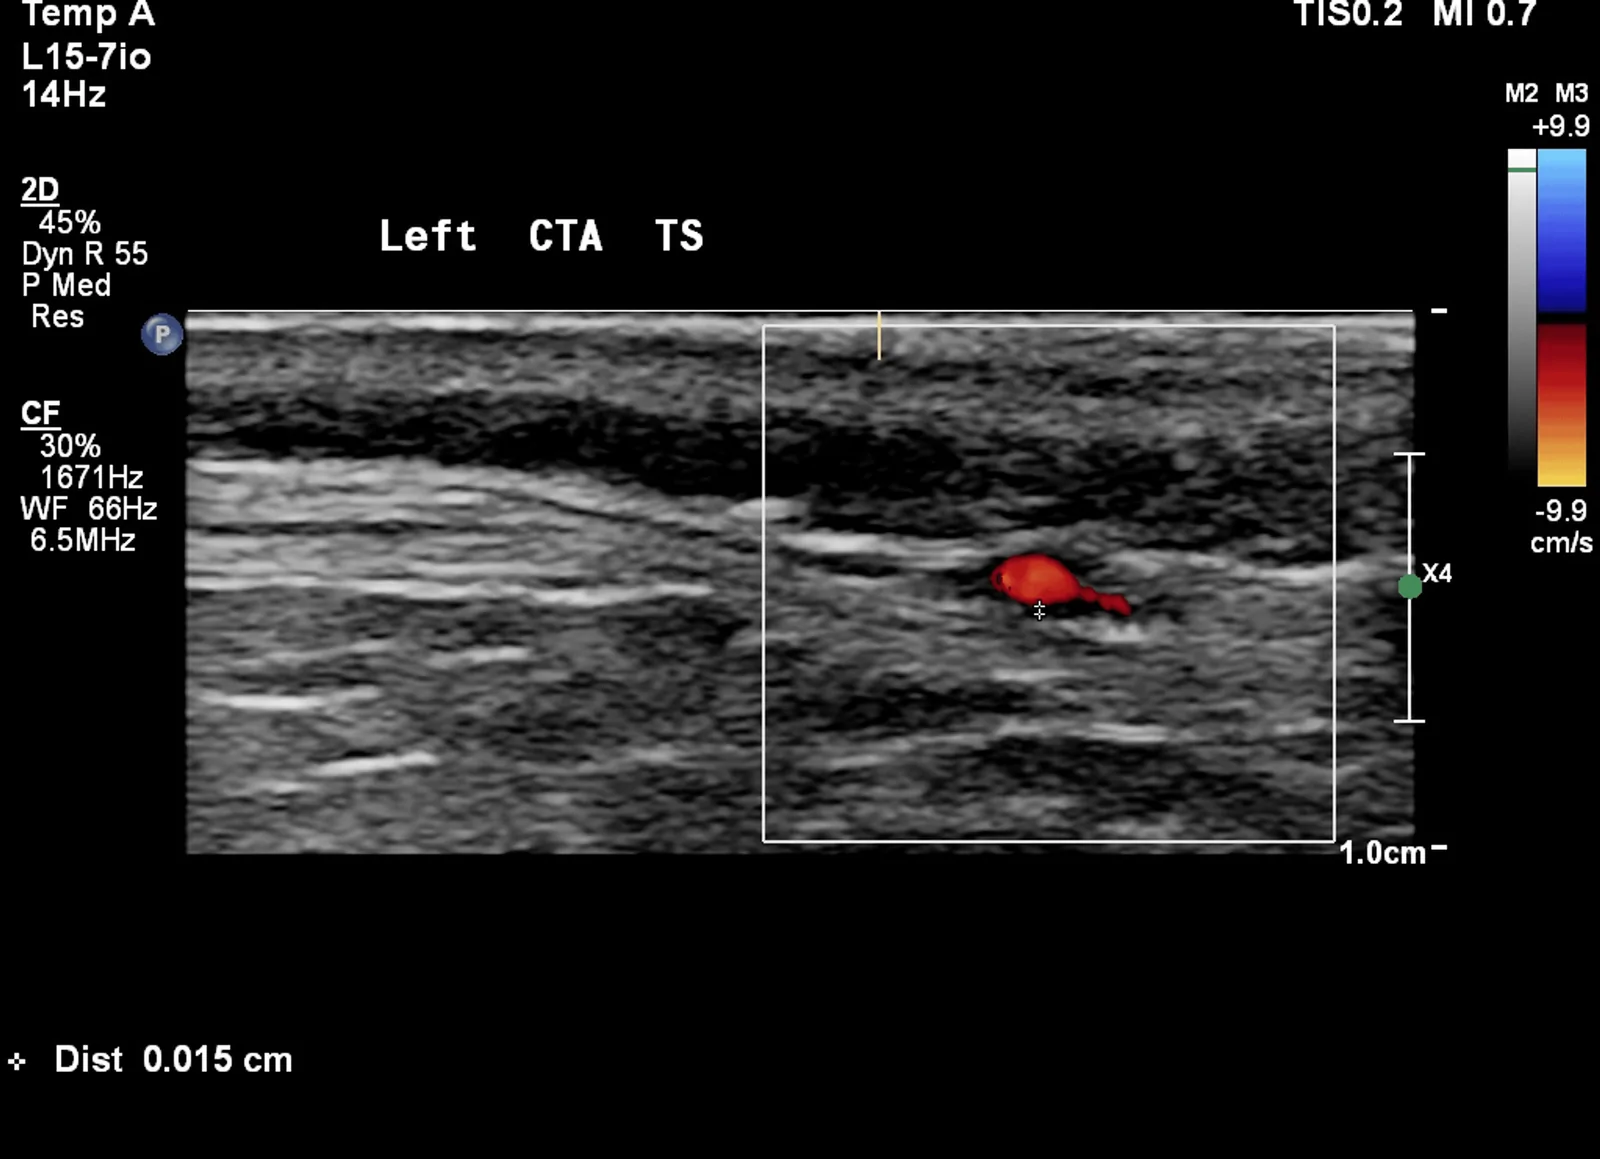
Halo sign demonstrated on temporal artery ultrasound in red
